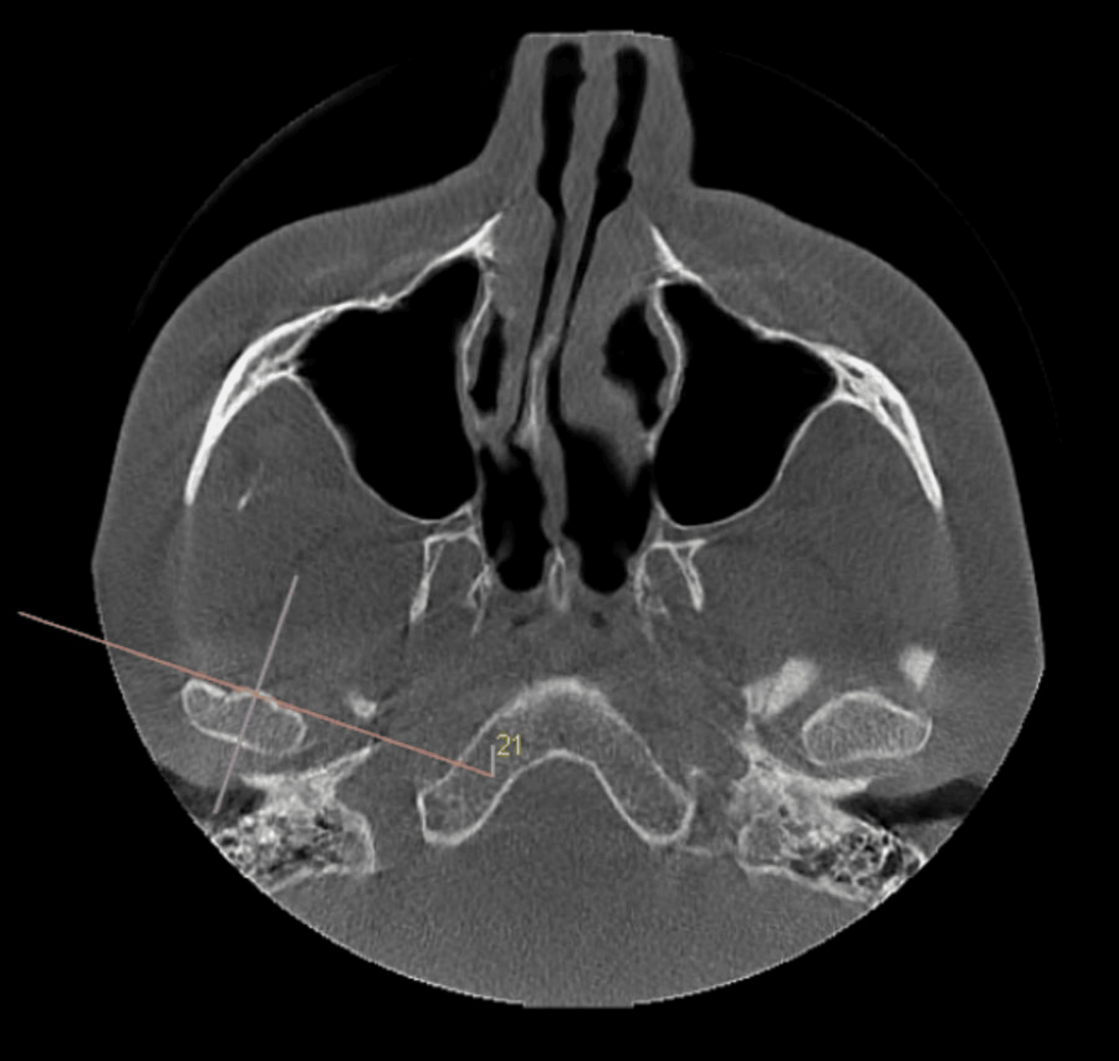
Axial slice of cone beam computed tomography (CBCT) image.
